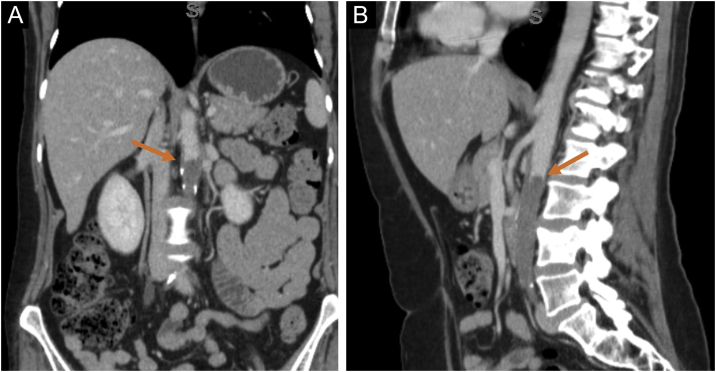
Computed tomography showing complete occlusion of the descending abdominal aorta (arrows). Coronal (A) and sagittal (B) scans.
